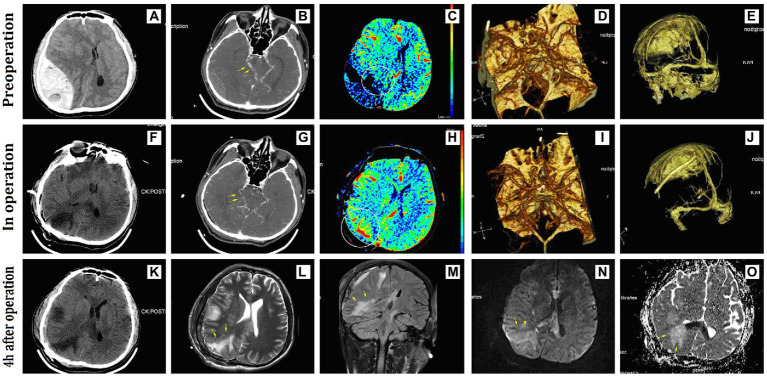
Representative radiographic images of Case 3. (A) A huge acute epidural hematoma occupied the right parietal-occipital area; (B–D) preoperative CTA showed compression and displacement of the right posterior cerebral artery; (C) preoperative CBF showed low perfusion due to hematoma compression in the right occipital lobe; (E–J) no significant compression on the venous sinus before and after surgery; (F–K) intra- and postoperative CT showed a low-density lesion in the right occipital lobe; (G–I) intra-operative CTA showed lessened displacement of the posterior cerebral artery; (H) intra-operative CBF showed increased perfusion in the right occipital lobe; (L–M) MRI examination performed 4 h after surgery showed long-signal shadows in the compressed areas of the right occipital lobe and corpus callosum on T2WI and FLAIR sequence; (N,O) the right occipital lobe showed long-signal on the DWI sequence and ADC sequence. CBF, cerebral blood flow; FLAIR, fluid-attenuated inversion recovery.
